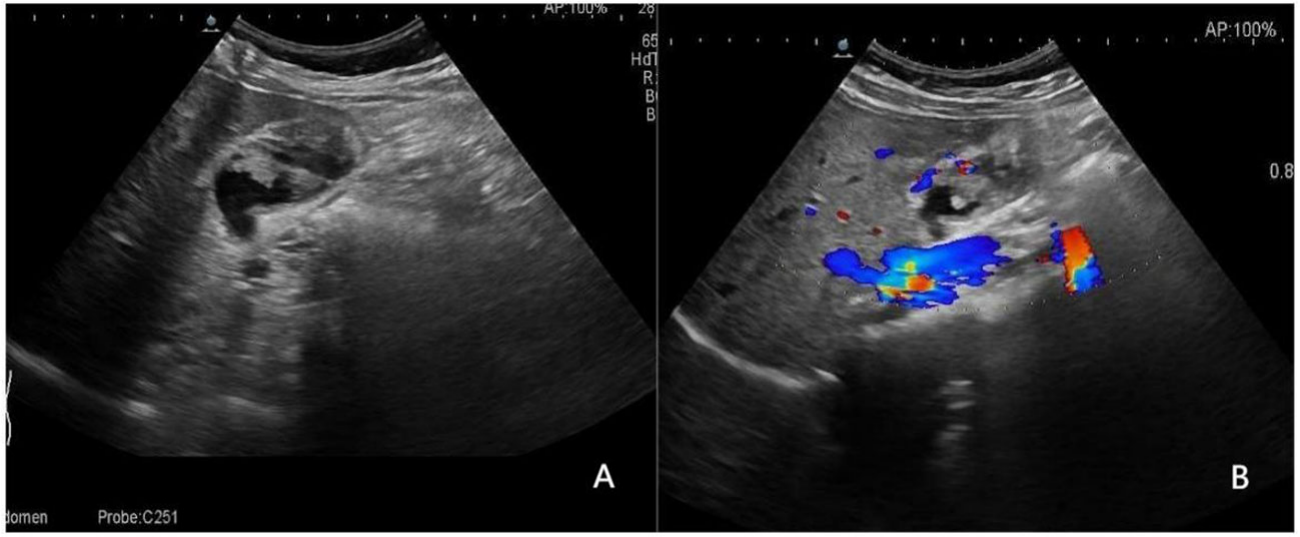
A 52-year-old man with a pathologically proven adenocarcinoma. (A) Focal thickening of the base of the gallbladder, with no stratified appearance, and loss of interface with the liver.; (B) CDFI=II. Based on a consensus review of the sonographic findings, the lesion was categorized as GB-RADS 4, GB-RADS+CDFI 4.
